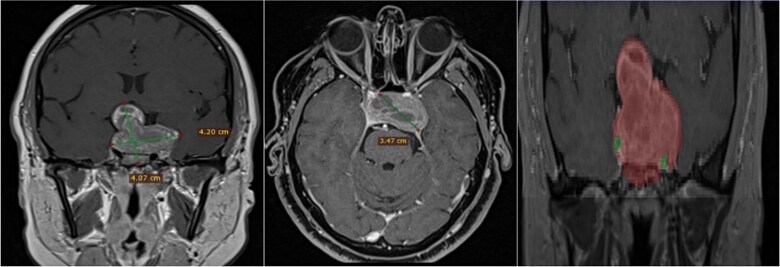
Contrast-enhanced T1 MRI of pituitary marcoadenoma. Multiplanar conventional geometric volumetry.
